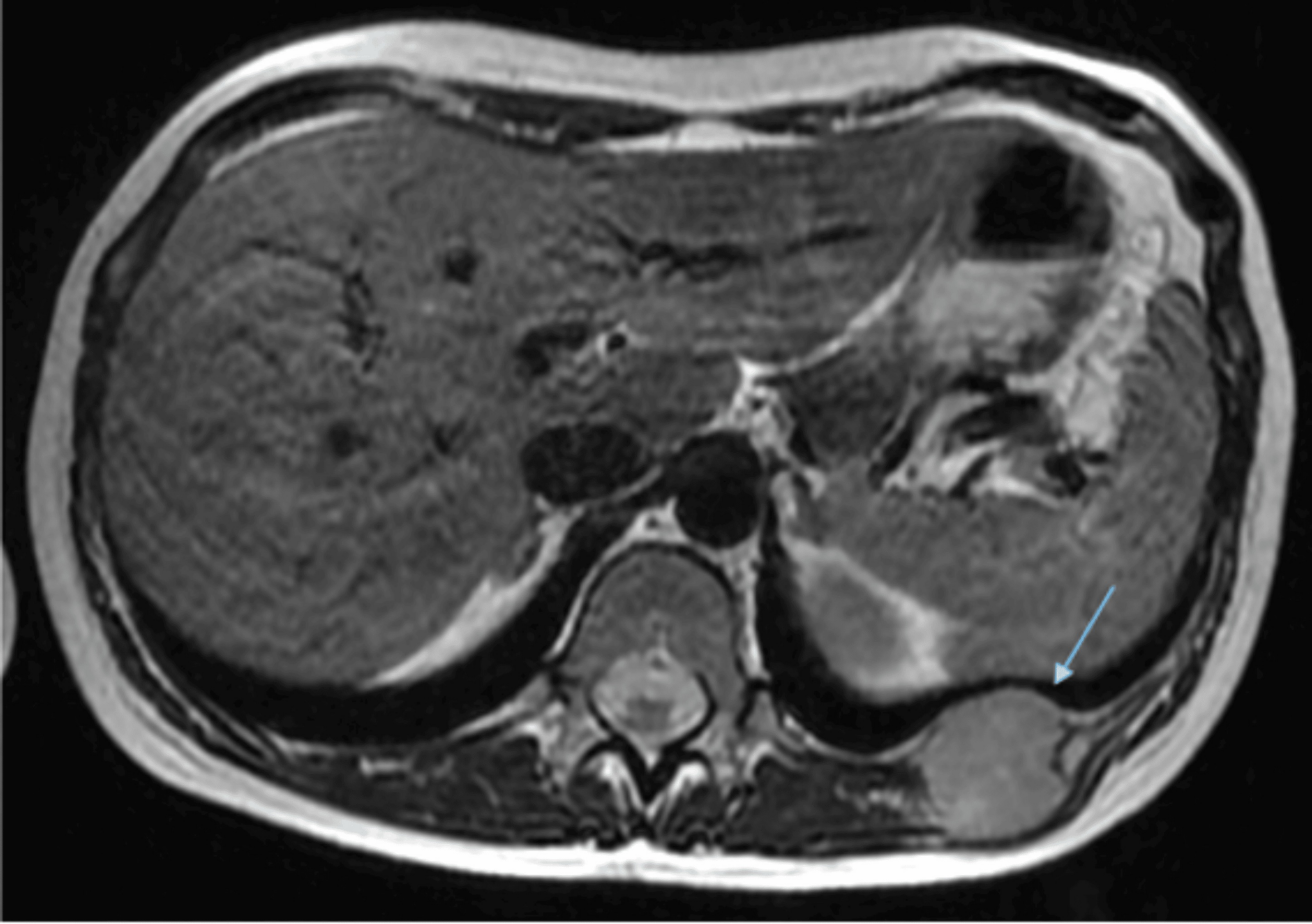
Thoracic MRI The blue arrow indicates a well-defined oval lesion located on the 11th left costal arch showing slight hypersignal (which points to the aggressiveness of the injury).
